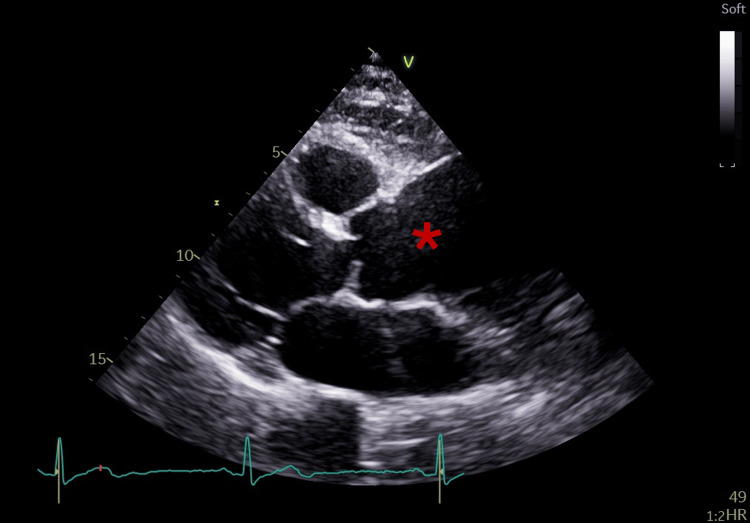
Transthoracic echocardiogram. Transthoracic echocardiography demonstrating a markedly dilated ascending aorta, consistent with ascending aortic aneurysm (*).
